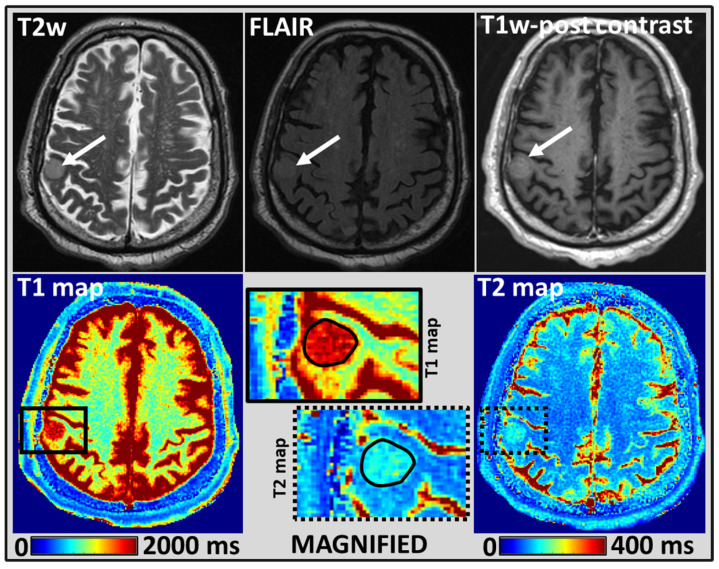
Representative MR images from a 72-year-old male patient with BM in the right parietal lobe treated with SRS and had a history of primary lung cancer: T2w (arrow pointing to the lesion), T2w fluid-attenuated inversion recovery (FLAIR), and T1w post-contrast images showing BM lesion at right parietal lobe (top row). MRF-derived quantitative T1 and T2 maps (bottom row). A black solid and dotted rectangle region on T1 and T2 maps, respectively, were magnified, and the placement of a manually drawn ROI for the BM lesion was shown in a solid black line. The MRF-derived T1 and T2 values at the BM lesion were 2355 ms and 154 ms, respectively.
